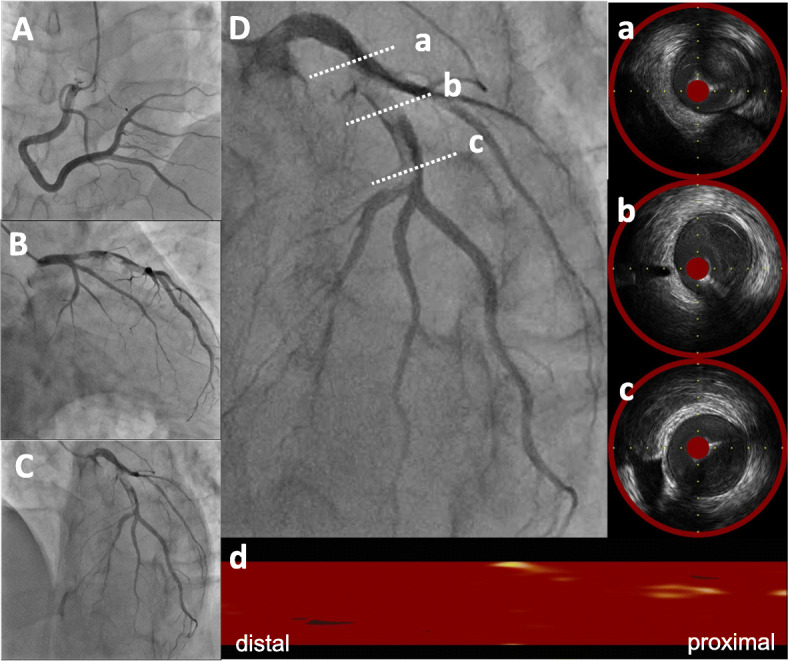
Baseline coronary angiography and near-infrared spectroscopy–intravascular ultrasonography. Coronary angiography (CAG) revealed severe stenosis from mid to proximal left anterior descending (LAD) artery and no severe stenosis in the right coronary artery and circumflex artery (A-C). Near-infrared spectroscopy–intravascular ultrasonography (NIRS–IVUS) findings in the culprit lesion showed a low echoic component suspecting thrombus continuing from mid to proximal LAD (D-a, b, c) and organic fibrous plaque behind the thrombus. The lipid plaque is shown as a yellow region on the chemogram. NIRS chemogram map presented the maximal lipid core burden index (4 mm) = 81 (D-d)
